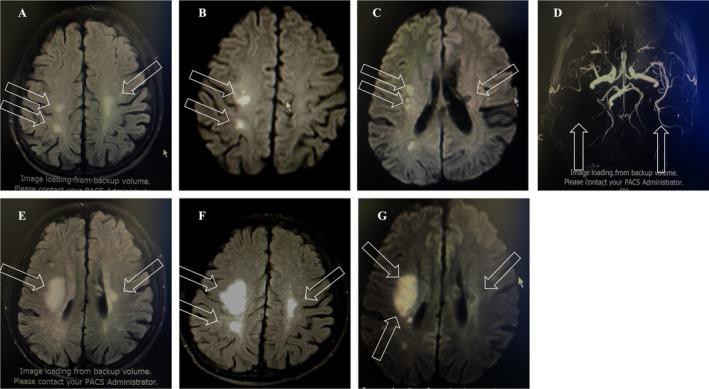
Neuroimaging features of the patient after two strokes. Axial T2‐FLAIR and DWI imaging of the patient with stroke. The patient demonstrated an acute stroke in the right parietal lobe and a remote stroke on the left centrum semiovale on the T2‐FLAIR window (A) and DWI imaging window (B, C) at the time of the first stroke. The MRA shows occlusion of the lower trunk of the right middle cerebral artery M2 segment, with a sparse representation of the distal branches of the right middle cerebral artery (D). The acute stroke on the right frontal and parietal lobes and remote stroke on the left centrum semiovale on T2‐FLAIR window (E) and DWI imaging window (F, G) at the second time of stroke. The arrow showed the lesions in each picture.
